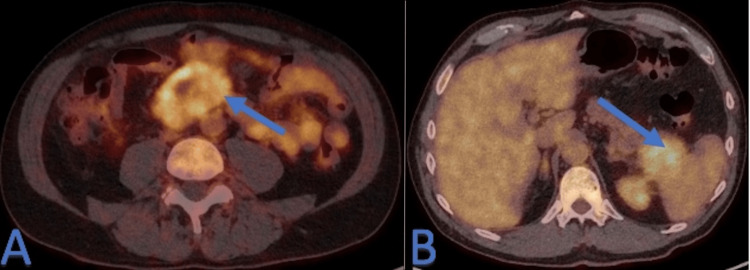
Initial F-fluorodeoxyglucose (FDG) positron emission tomography/computed tomography (PET-CT) We can observe the FDG uptake on both mesenteric (arrow) on A and pancreatic mass (arrow) on B.
